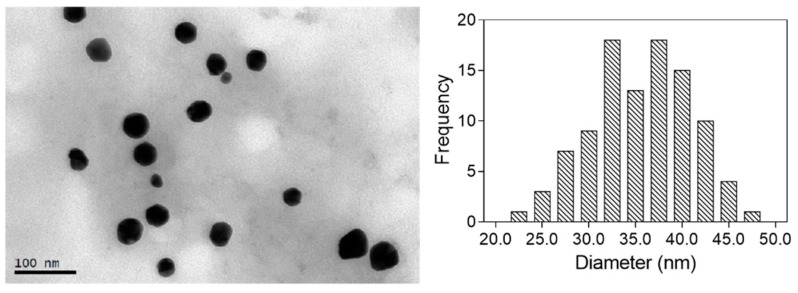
TEM image of Au@CT with its corresponding size distribution histogram.
